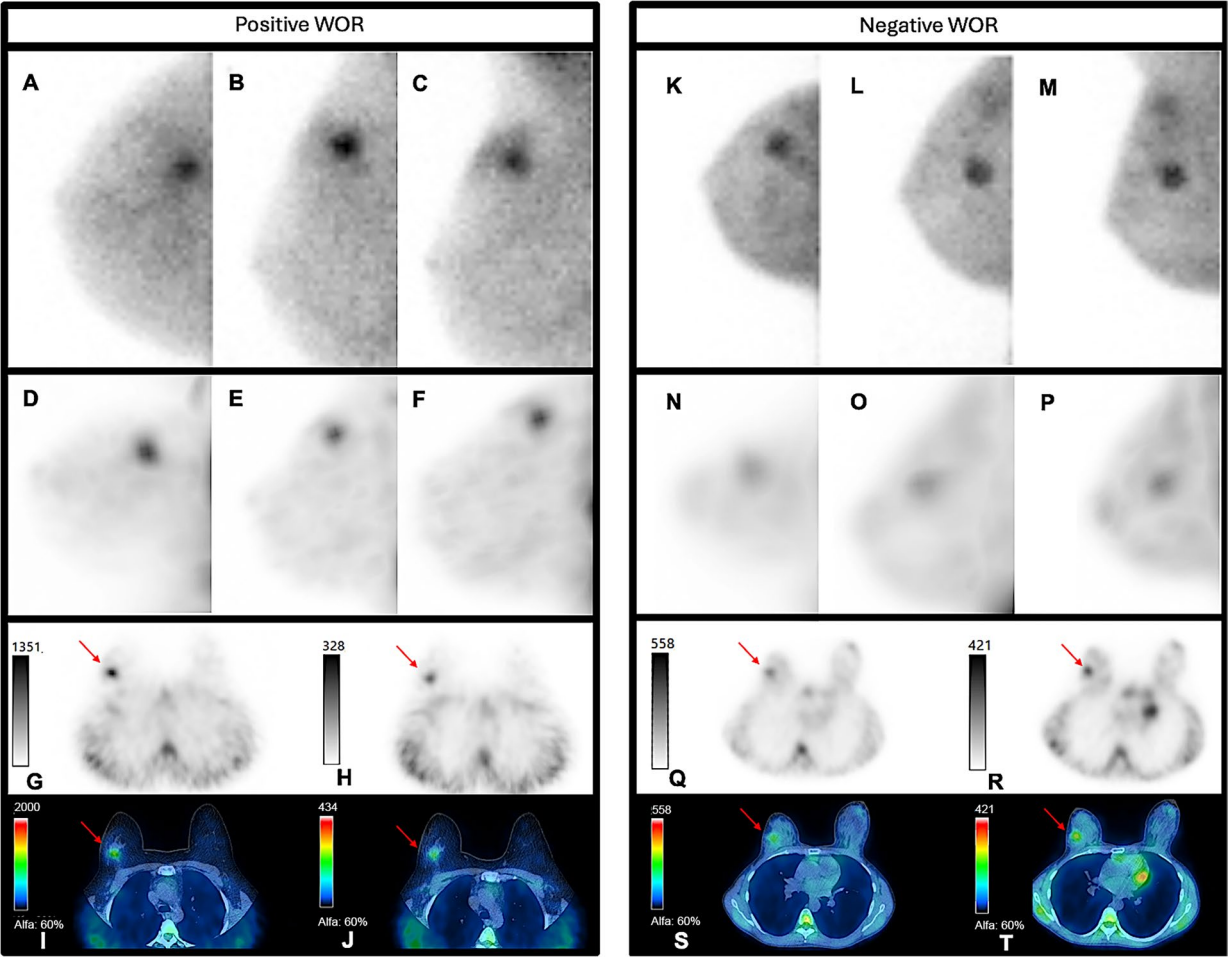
Molecular breast imaging (MBI) and single-photon emission computed tomography combined with computed tomography (SPECT/CT) images of two patients. The first patient (A to G) is a 45-year-old woman with invasive ductal carcinoma grade 3, estrogen receptor-negative, progesterone receptor-negative, and HER2-negative (triple-negative), MBI and SPECT/CT show high focal [^99m^Tc]Tc-sestamibi uptake of a 2 cm in diameter tumor located in the lateral upper quadrant of the right breast (red arrow) with visual lower uptake on delayed SPECT/CT and wash-out rate (WOR) of 18. The second patient (K to T) is a 43-year-old woman with invasive ductal carcinoma grade 3, estrogen receptor-positive, progesterone receptor-positive, and HER2-negative, MBI and SPECT/CT show moderate focal [^99m^Tc]Tc-sestamibi uptake of a 2 cm in diameter tumor located in the lateral upper quadrant of the right breast (red arrow) and visual increased uptake on the delayed images (WOR -33). MBI right craniocaudal view (A and K), MBI right lateral oblique view (B and L), MBI right mediolateral view (C and M), SPECT maximum intensity projection (MIP) right craniocaudal view (D and N), SPECT MIP right lateral oblique view (E and O), SPECT MIP right mediolateral view (F and P), axial SPECT of early (G and Q), and delayed (H and R) acquisition, fused axial SPECT/CT images of early (I and S) and delayed (J and T) acquisition.
